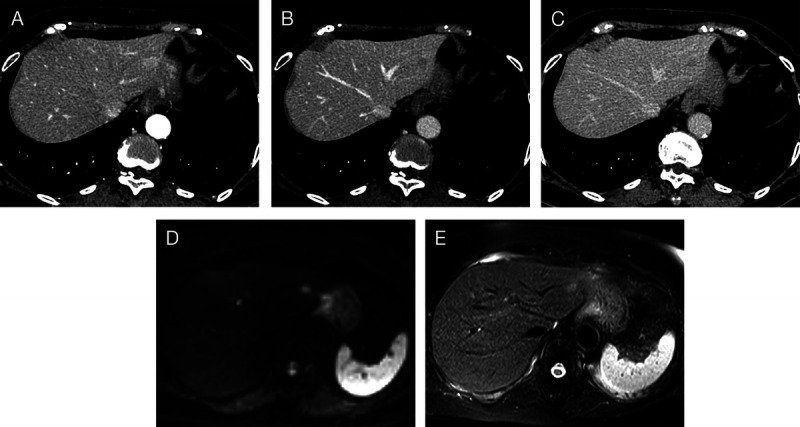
Contrast-enhanced computed tomography shows homogeneous enhancement in the arterial dominant phase (A) and wash-out with suspicion of slight perinodular enhancement in the portal (B) and late phases (C). Magnetic resonance imaging shows high-signal intensity on diffusion-weighted imaging (D) and slight signal intensity on T2-weighted imaging (E).
